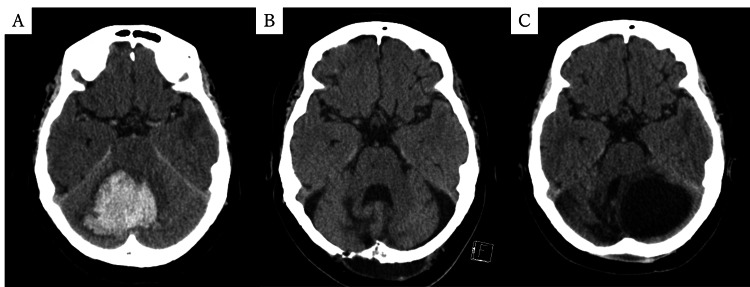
Serial CT images (A) Initial CT showing cerebellar hemorrhage. (B) CT at one month postoperatively, without cyst formation. (C) CT at eight months postoperatively demonstrating a newly developed cystic lesion in the left cerebellar hemisphere.
